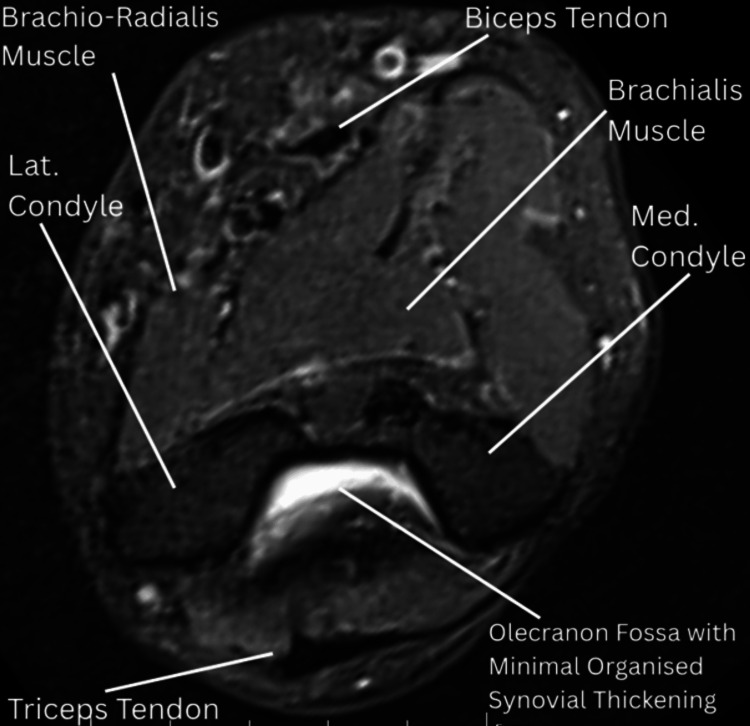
MRI (post-treatment): axial proton density fat-suppressed (PDFS) image demonstrating a marked reduction in diffuse synovial thickening, with only minimal organized residual synovial tissue remaining.
